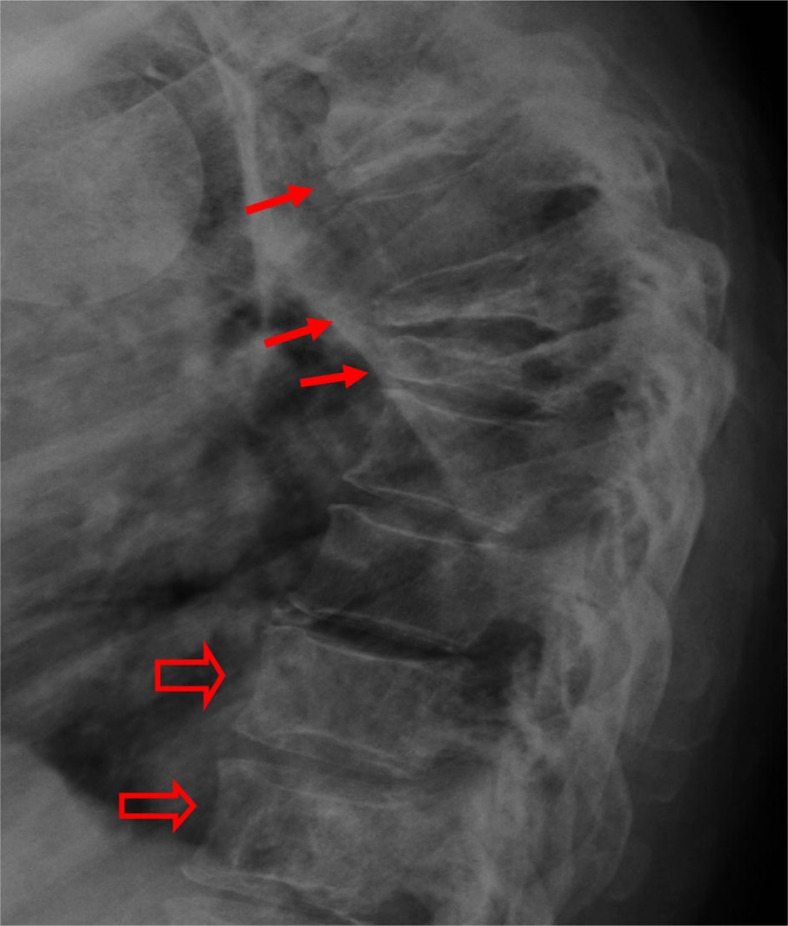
A lateral thoracic spine radiograph shows multiple severe vertebral body compression fractures (small arrows), vertebra plana, also characteristic of multiple myeloma. At T11 and T12 (open arrows), mild vertebral sclerosis is a result of treatment and healing.
